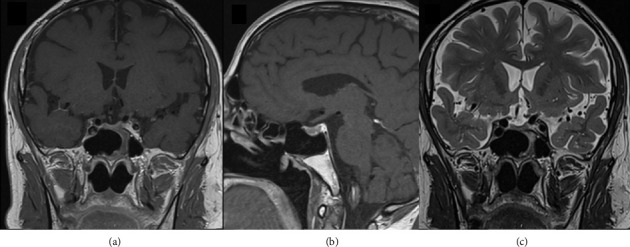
Postoperative MRI brain scan 9/2023. (a) Coronal T1-weighted image shows less compression of the left sinus cavernous. (b) Sagittal T1-weighted image shows relief of pressure on the optic chiasm. (c) Coronal T2-weighted image shows cessation of the preoperative cystic compartment.
